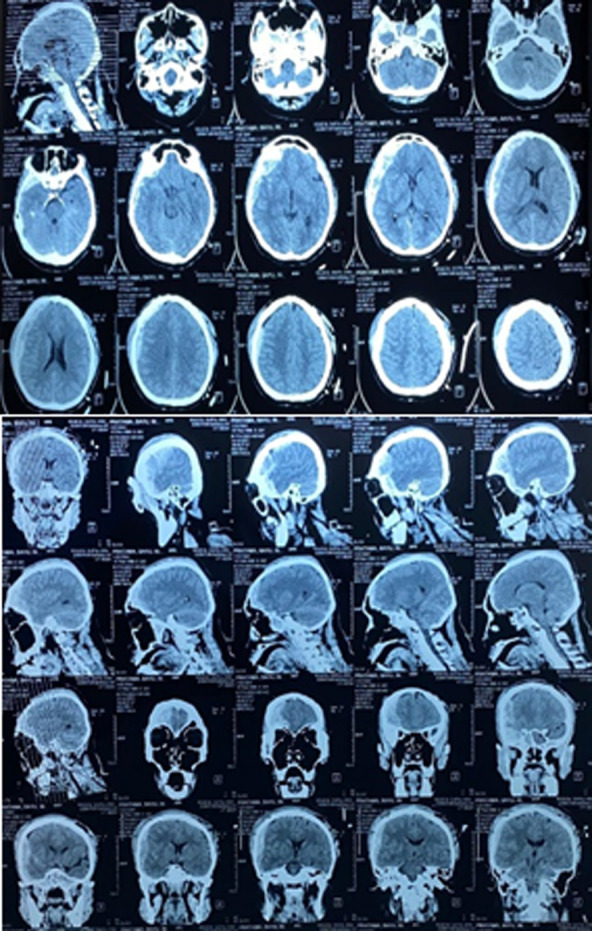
head CT before surgery showing Right frontal and temporal epidural hematoma and coronal suture diastasis
